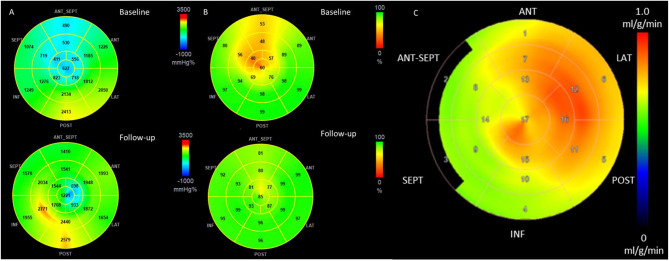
Evaluation of myocardial work indices and perfusion in patient with acute myocardial infarction polar maps showing echocardiographic myocardial work indices (A and B) and resting myocardial blood flow (C) assessed by 15-oxygen water positron emission tomography (PET) in a patient with acute myocardial infarction caused by occlusion of the proximal left anterior descending coronary artery. Myocardial work index (A) and myocardial work efficiency (B) Are reduced in the anteroseptal region at baseline (upper panels) and show partial recovery at the 6-month follow-up (lower panels). Myocardial blood flow is reduced in the anteroseptal region. Please note different orientation of echocardiographic and PET polar maps
